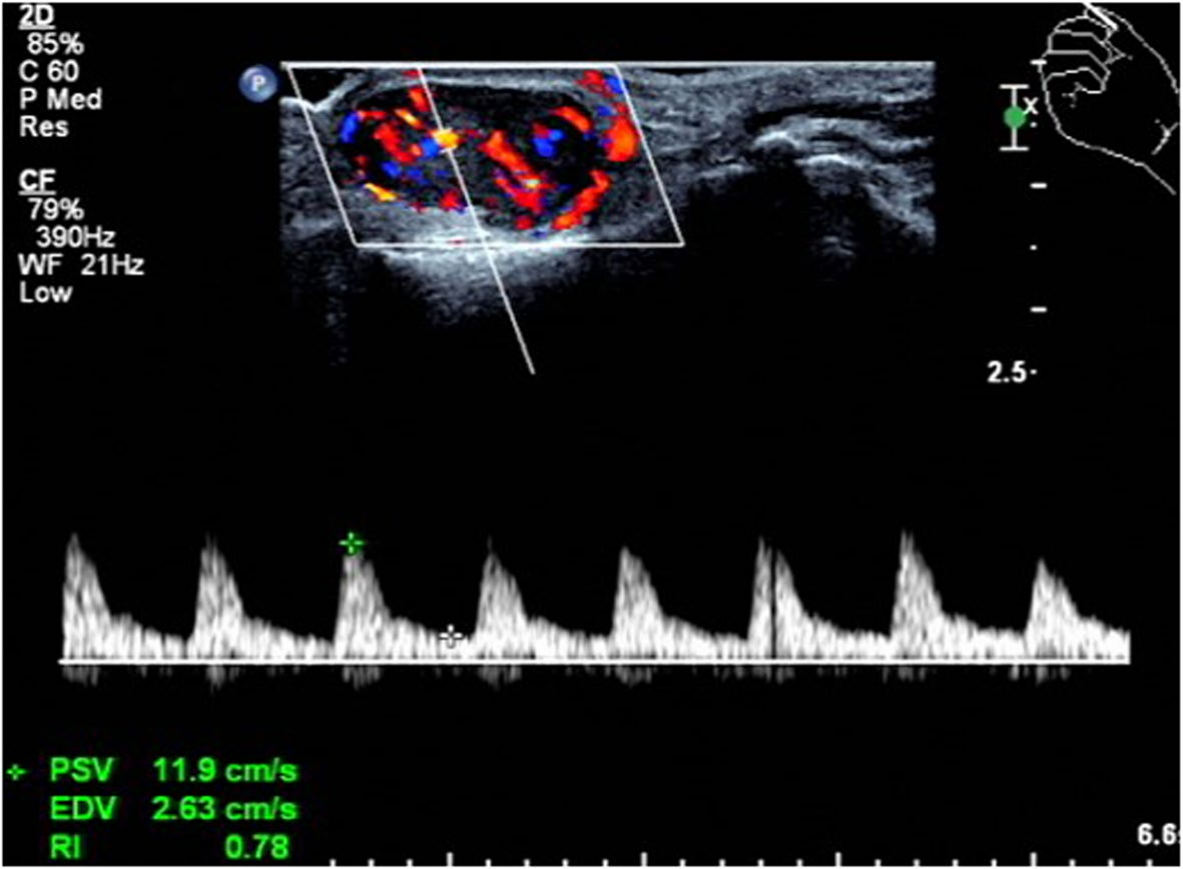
A 67-year-old man with a painless mass on the radialis surface of the wrist. Sonography shows a homogeneous hypoechoic nodule with abundant blood flow (Class III) and the arterial flow spectrum is detected
